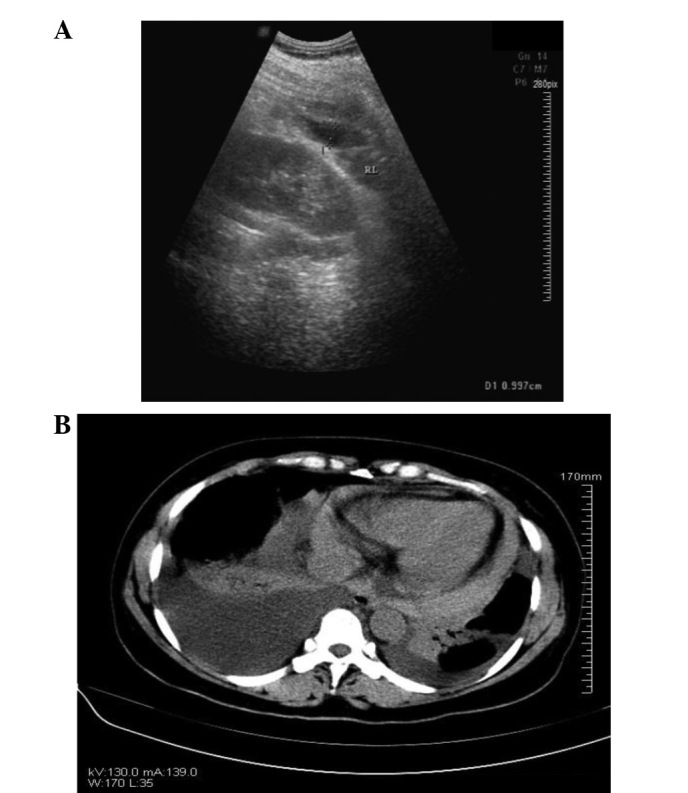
(A) Bedside B-mode ultrasound showing moderate amounts of bilateral pleural effusion and a small amount ofpericardial effusion. (B) CT indicating bilateral pleural and pericardial effusions.
